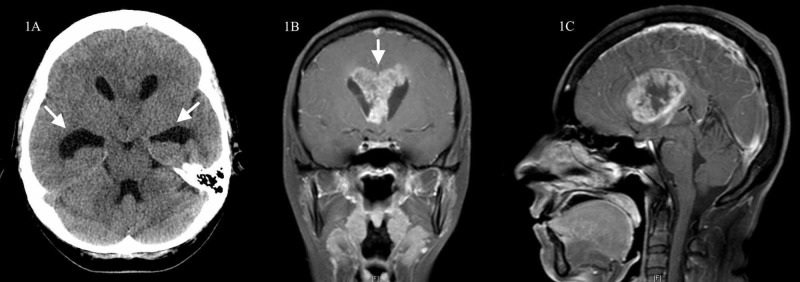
CT head without contrast and post-contrast T1-weight MRI brain showing hydrocephalus with an associated enhancing midline mass CT head without contrast shows hydrocephalus with remarkable dilation of the temporal horns from a midline structure obstructing the foramina of Monro (Figure 1A, left). Post-contrast coronal and sagittal T1-weighted image MRI of the brain shows an enhancing mass involving the septum pellucidum and corpus callosum with a necrotic component (Figures 1B, center; 1C, right). CT, computed tomography; MRI, magnetic resonance imaging.
